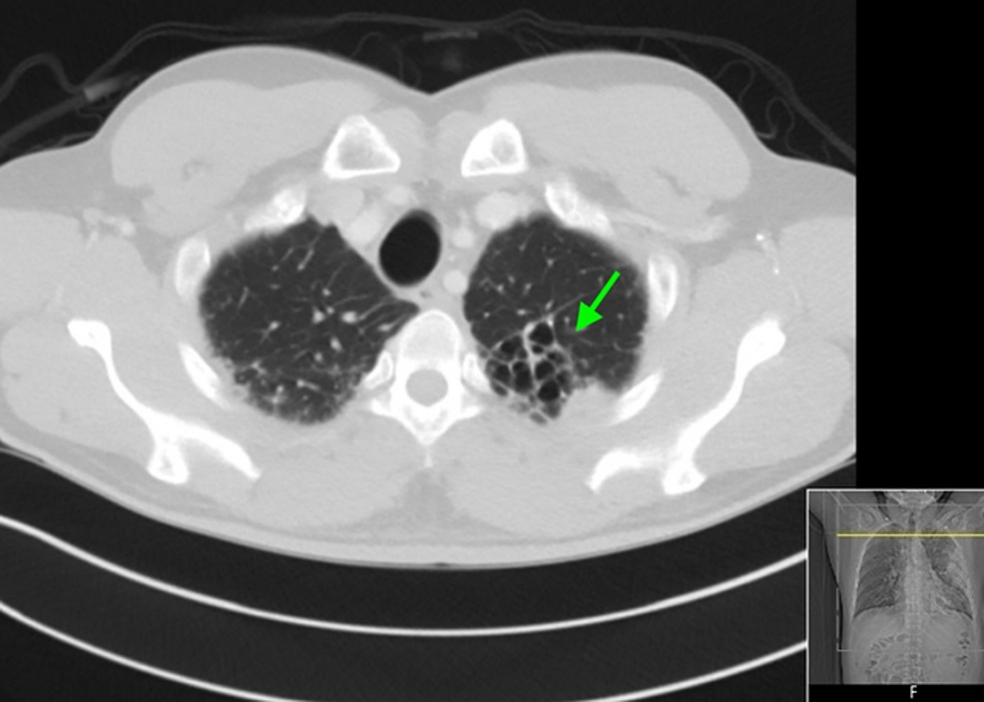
CT of the chest - image 1 The image shows evidence of mediastinal adenopathy and cystic bronchiectasis in the left upper lobe with calcification suggesting old granulomatous disease (arrow) CT: computed tomography
